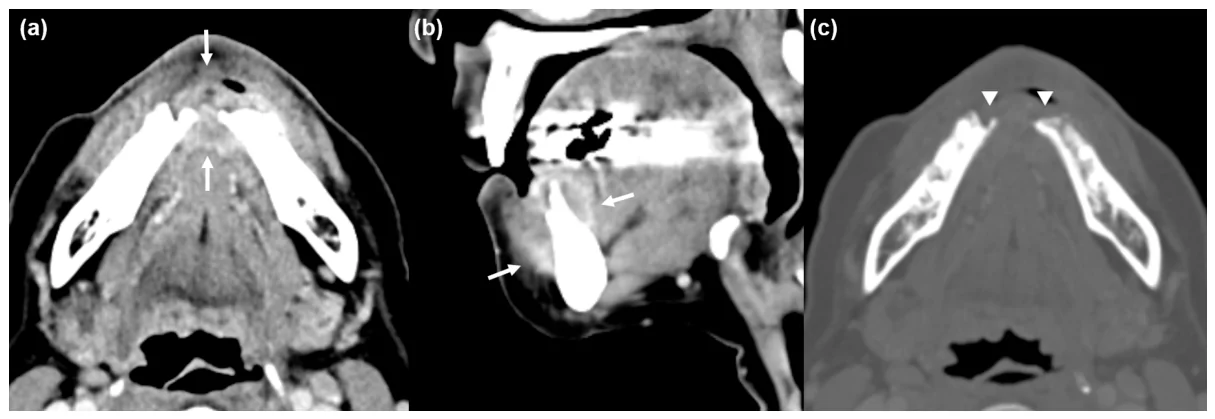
CT findings. (a) Axial contrast-enhanced CT image shows a heterogeneously enhancing soft-tissue gingival mass at the mandibular symphysis with focal extension to the anterior floor of the mouth (arrows). (b) Sagittal contrast-enhanced CT image shows bidirectional tumor extension across the mandible (arrows). (c) Axial bone-window CT images demonstrate cortical bony destruction of the mandible (arrowheads).
